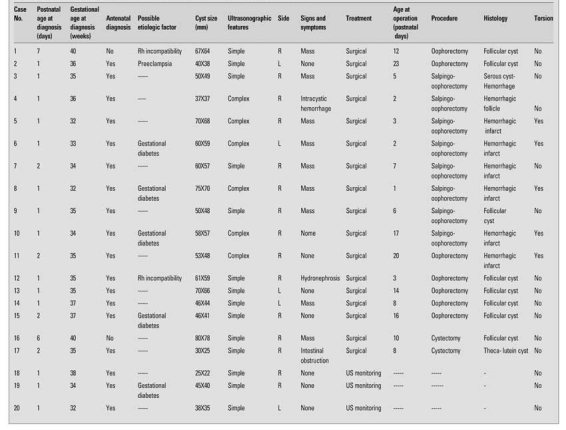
Demographic, clinical, ultrasonographic and other features of our patients with ovarian cysts
